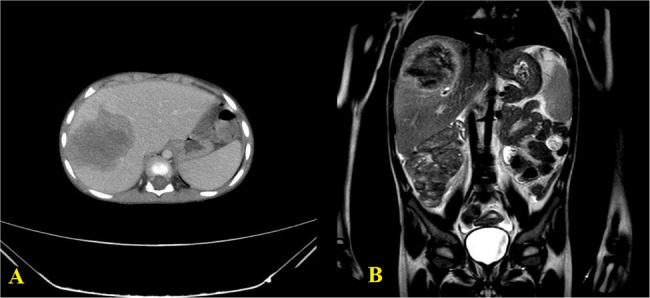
(A) Portovenous phase of axial CT scan with contrast shows liver mass. (B) Coronal T2W image of the same liver mass
